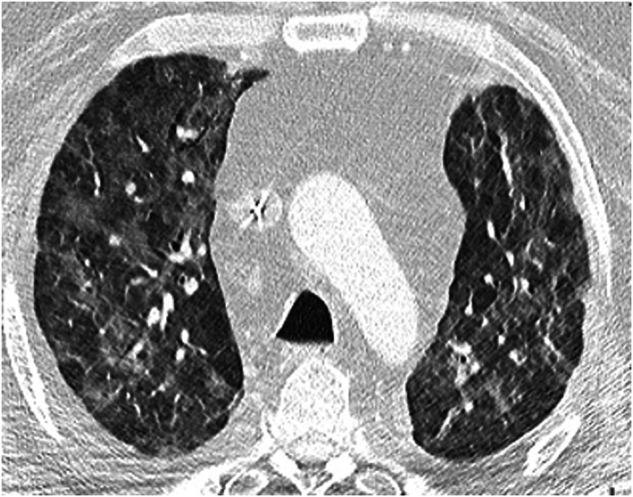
CT scan of a patient with systemic anticancer therapy-induced interstitial lung disease with the hypersensitivity pneumonitis pattern, diagnosed by multidisciplinary team consensus. The examinations were performed with contrast to exclude the presence of pulmonary embolism. Diagnosis of the three-density pattern is therefore difficult after injection. It is recommended that the examination be repeated without contrast agent if there is any uncertainty. This CT scan depicts mosaic density with extensive ground glass opacities and exclusion of several secondary lobules. Images reproduced with permission from Dinkel J. 2023. University Hospital LMU Munich, Munich, Germany
